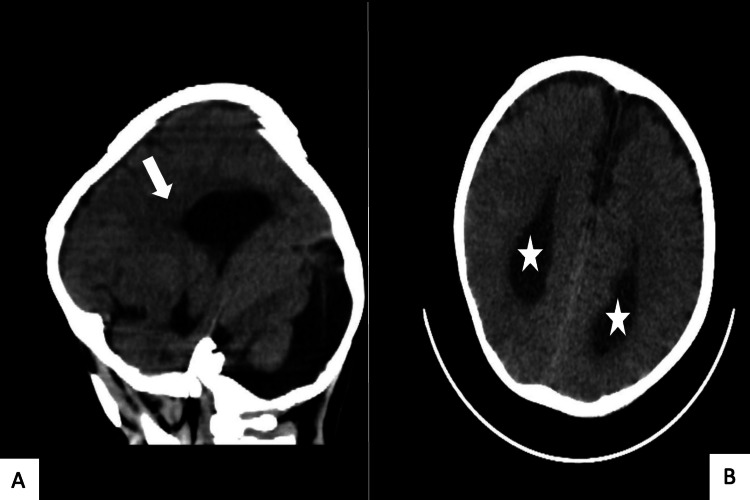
Brain computed tomography without contrast. Panel A: Suggestive of corpus callosum agenesis (white arrow). Panel B: Enlargement of lateral ventricular (white star).
